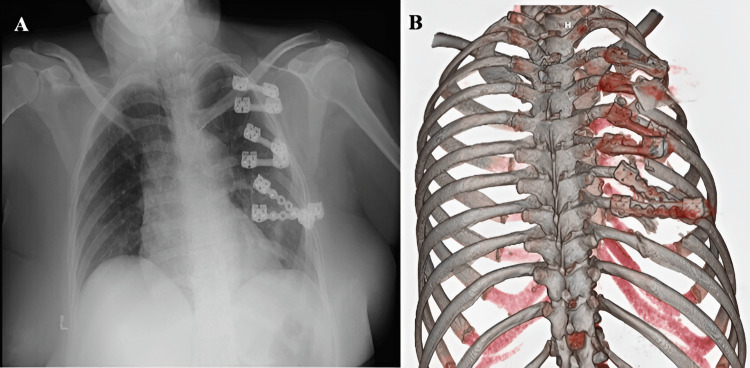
A: Chest radiograph showing evidence of displaced rib fixation devices; B: Chest computed tomography with 3D reconstruction demonstrating in greater detail the displacement of the rib fixation devices
